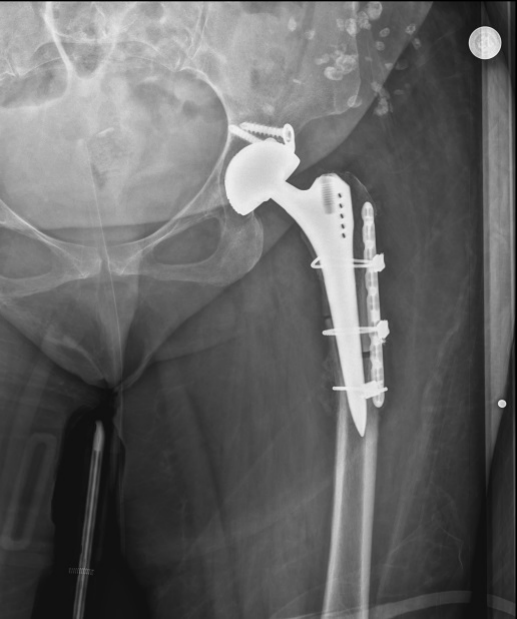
Early postoperative X-ray radiograph of patient who underwent osteotomy fixation with plate and cable.
